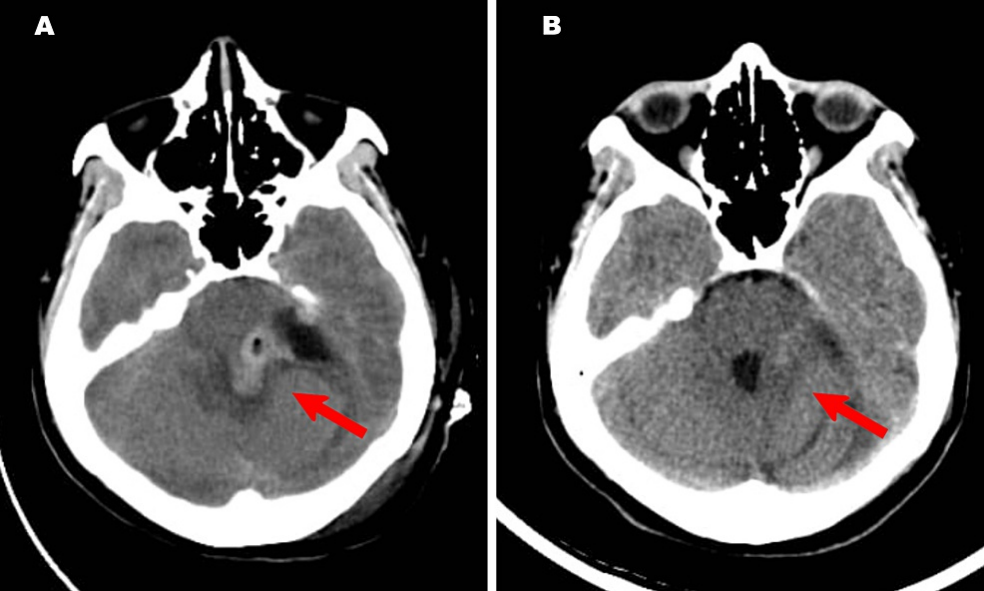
Postoperative CT scan images A- Immediate postoperative non-contrast axial CT scan showing tumour decompression; B- Three-month follow-up non-contrast CT scan showing good clearance of the lesion. Red arrow identifies the operative field.
